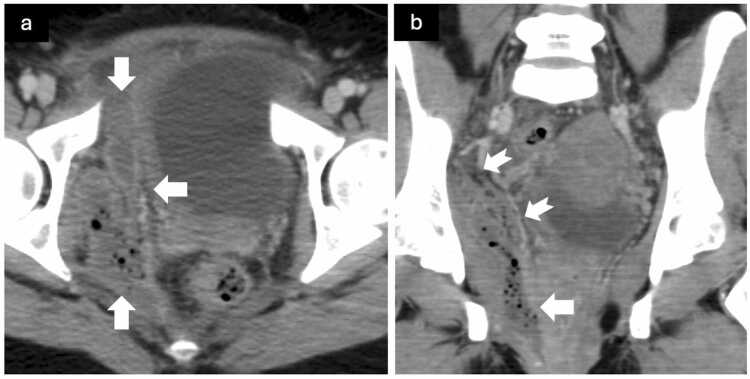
30year – old female with prolonged labor and instrumented vaginal birth presenting 2 weeks postpartum with sepsis. Contrast enhanced CT pelvis (a) axial and (b) coronal images show a right paravaginal mixed density complex fluid and gas containing multiloculated collection (bold arrows) consistent with infected vaginal hematoma with supralevator extension (notched arrows). DIAGNOSIS: postpartum haemorrhage and secondary infection.
